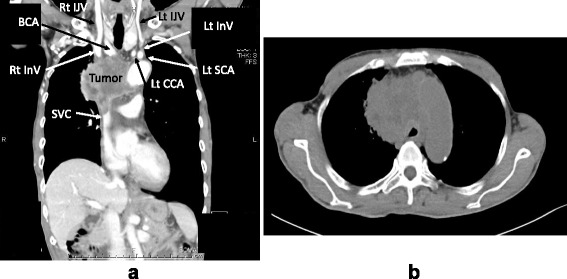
Contrast-enhanced CT findings. a A coronal slice showing a large mass (maximum diameter, 72.25 mm) in the mediastinum, which completely interrupts bilateral innominate veins. b An axial slice showing that the mass compresses the trachea with significant reduction of the tracheal cross sectional area by approximately 50 %. InV; innominate vein, IJV; internal jugular vein, BCA; brachiocephalic artery, CCA; common carotid artery, SCA; subclavian artery, SVC; superior vena cava
